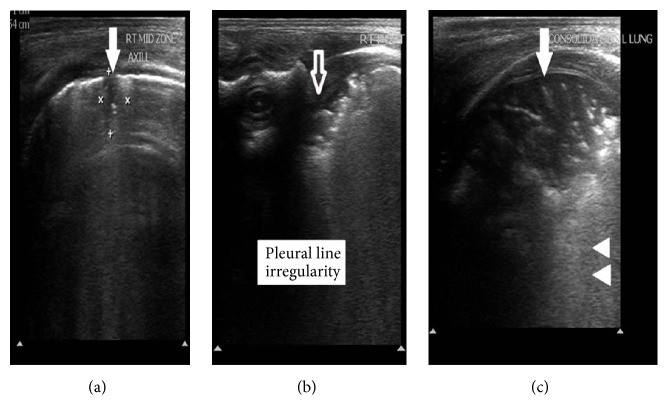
The LUS findings associated with VAP. Superficial high resolution lung ultrasound was done and images obtained in midaxillary (a), scapular (b), and midclavicular (c) lines at transverse and longitudinal planes. Multiple peripheral hypoechoic areas are seen which assume subpleural location showing irregular borders and air-bronchogram inside, suggesting pneumonic consolidations (solid arrows). Also thickened irregular pleural borders (empty arrow) with absent A-lines and coalescent B-lines around the consolidations (arrow heads).
